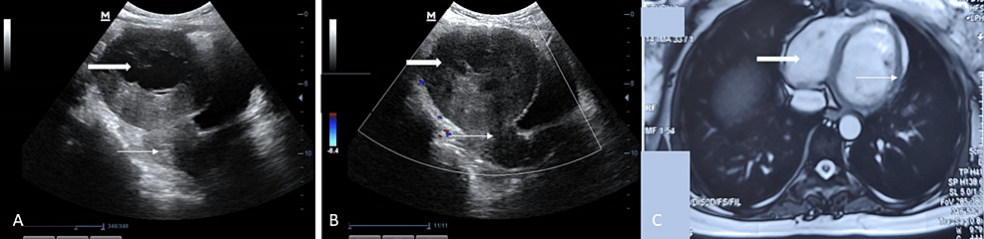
Pre-operative imaging A. 2D ultrasound showing a normal-sized uterus (thin arrow) with a solid lesion of size 8x7 cm with central degeneration (thick arrow) present superior to the uterus, suggestive of subserous uterine fibroid B. Color Doppler ultrasound showing the large hypoechoic solid mass with anechoic spaces and no internal vascularity (thick arrow). The bridging vascular sign with the uterus was absent (thin arrow), suggestive of the non-uterine origin of the mass, presumably a large gastrointestinal stromal tumor. C. MRI showing T2 hyperintensity suggestive of cystic degeneration (thick arrow), with a band of T2 hypointensity (thin arrow) separating the tumor from the uterus on all planes, characteristic of an ovarian fibroma.
